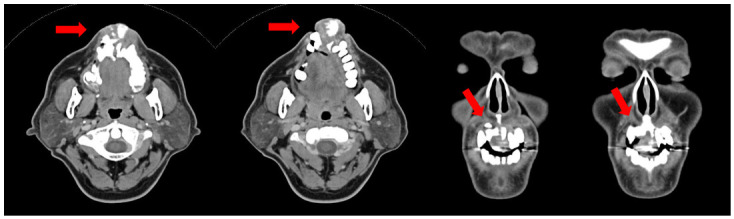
CT images suggested a periosteal or periodontal origin with minimal bone involvement.
